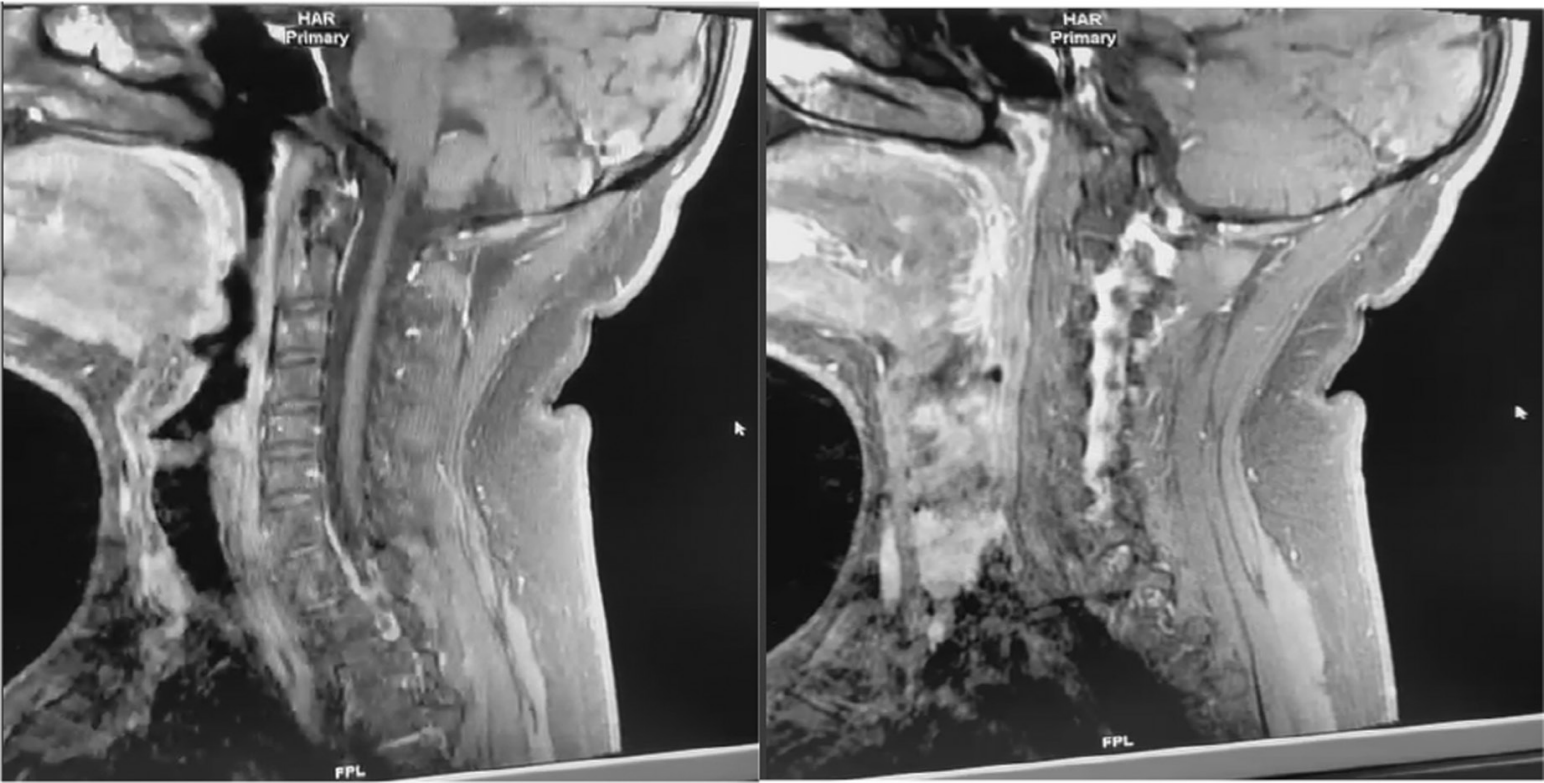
Cervical MRI with gadolinium injection, showing no enhancement of the lesions.
